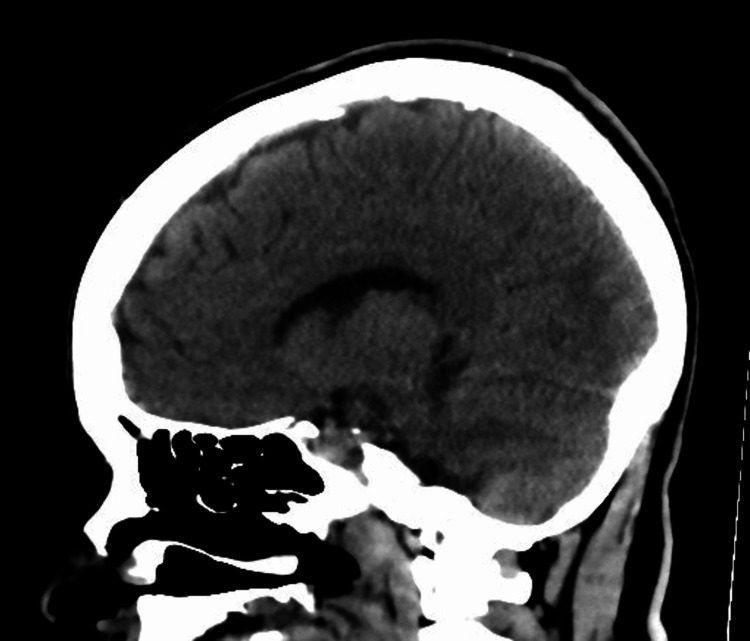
Brain CT scan (sagittal view) showing no evidence of intracranial hemorrhage, or focal mass lesions.
